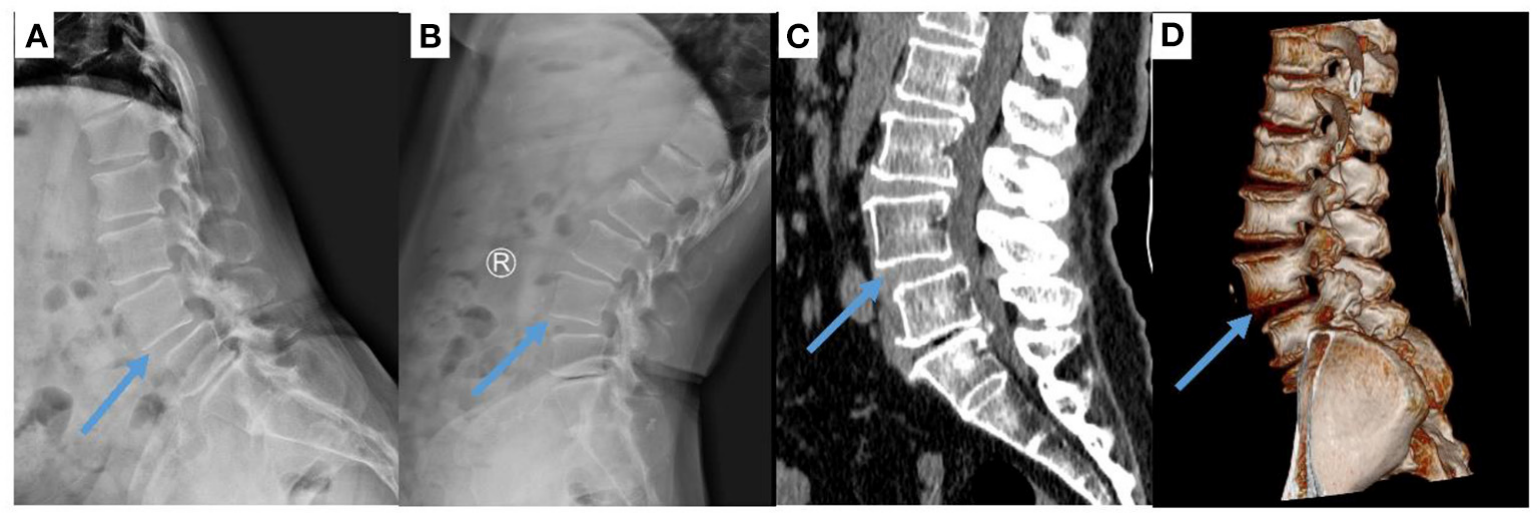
Image of L4/5 spondylolisthesis in a 65-year-old woman. (A,B) X-ray and (C,D) CT images show that the L4/5 vertebral body has slipped forward.
